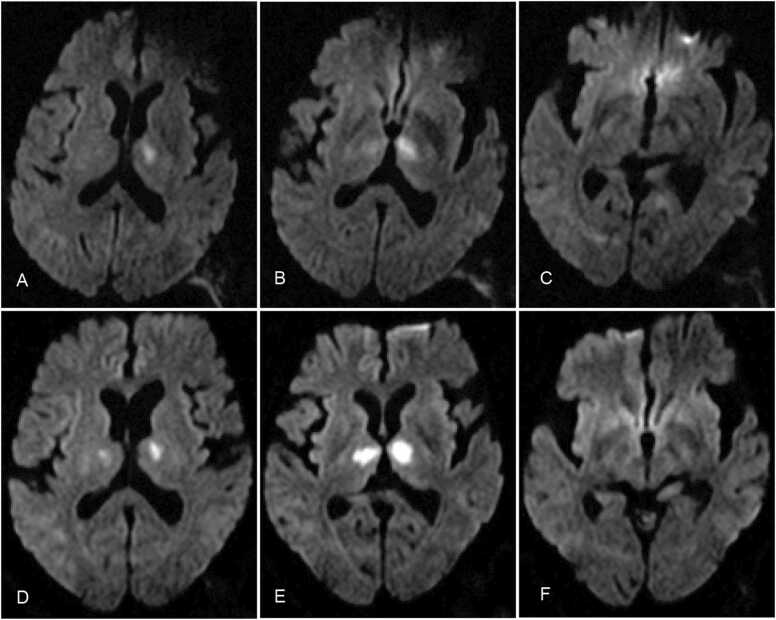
Diffusion-weighted MRI scans of Case 3 patient with AOP infraction. Diffusion weighted imaging A–C has shown asymmetric restricted diffusion in bilateral paramedian thalamus without midbrain 2 h after onset; D–F showed high signal intensity in the bilateral paramedian thalamus on DWI on the second day after onset.
